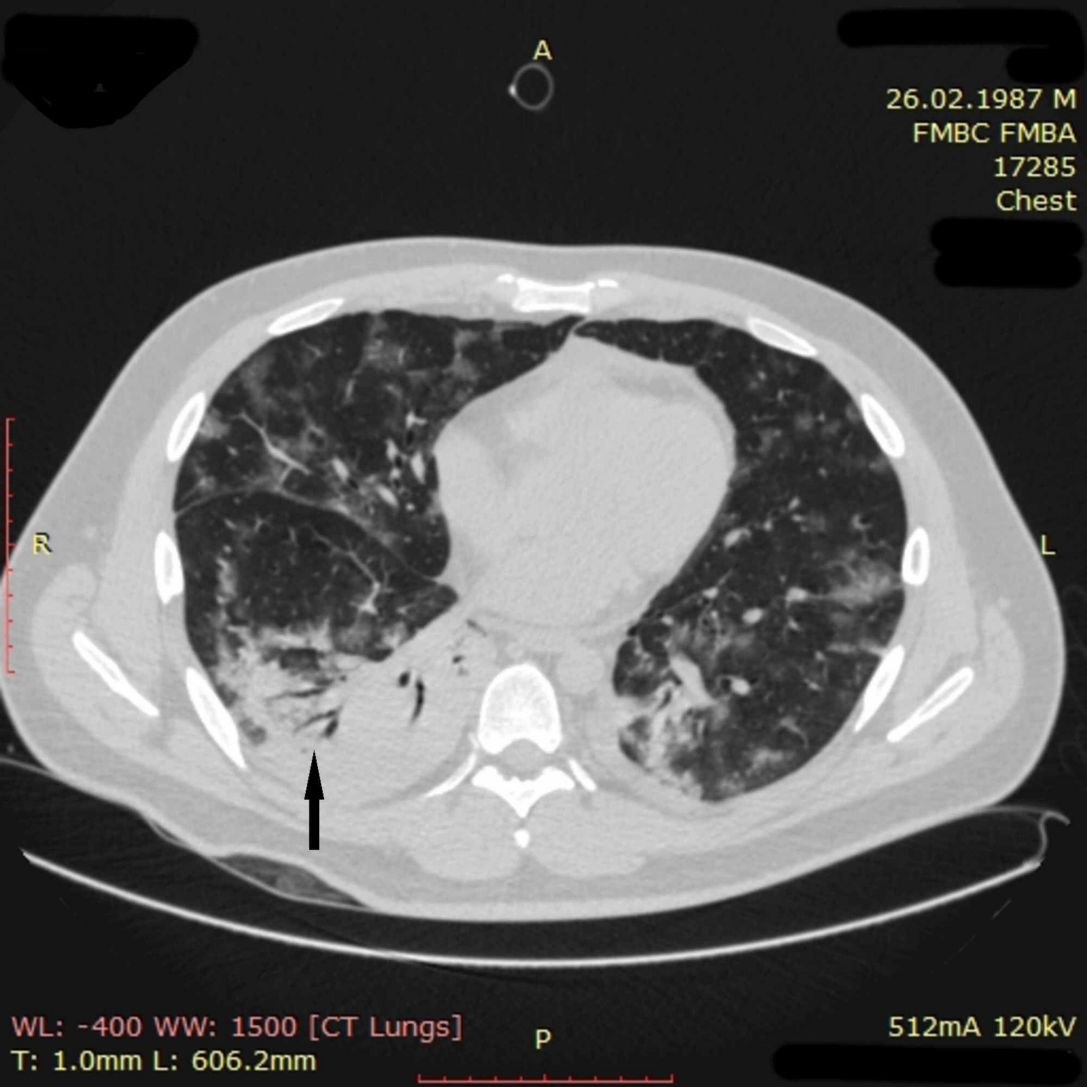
Chest CT imaging of patient 3 on day nine Chest CT performed on day nine showed a distinct positive change in CT picture compared to that of day four. The ground-glass opacities noticeably decreased in amount and density all over the lungs. Pneumatized parenchyma could be seen again in all pulmonary segments. The total volume of affected lung tissue was down to approximately 50%. However, a new zone of pulmonary tissue consolidation with a symptom of air bronchogram (black arrow) was found in the lower lobe of the right lung, suggestive of pneumonia CT: computed tomography
